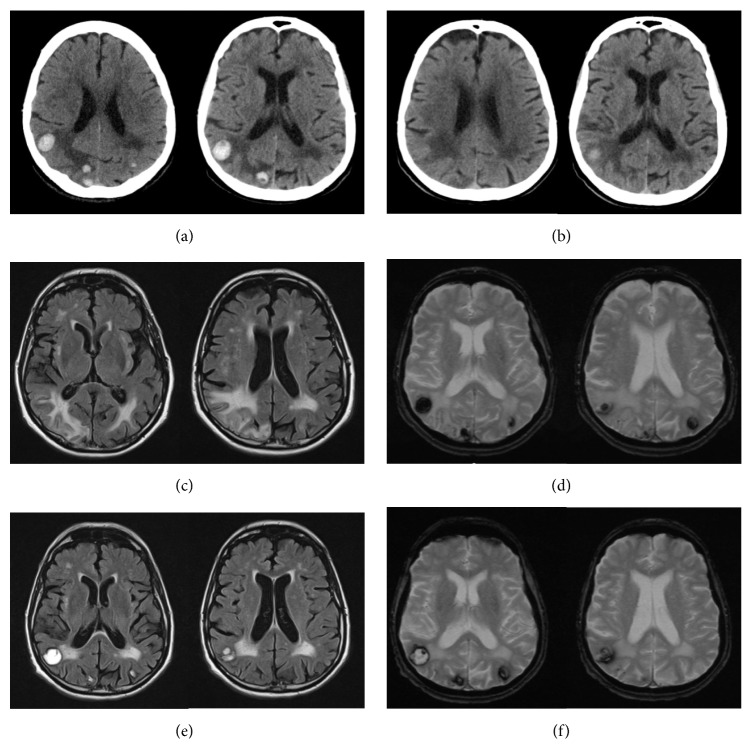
Patient 1: noncontrast brain CT-scan showing occipital bilateral hyperdense nodules, predominantly on the right side, surrounded by edema, corresponding to brain hematomas (a). Noncontrast brain CT-scan 18 days later showing regression of the size and number of hematomas with a decrease in the degree of the surrounding edema (b). MRI-scan 6 weeks later, axial Fluid Attenuated Inversion Recovery, FLAIR (c), and Gradient Echo, GRE (d), weighted sequences showing a marked susceptibility effect confirming the haemorrhagic lesions and the presence of surrounding edema. Follow-up MRI-scan 4 months later with same sequences, showing regression of the size and number of hematomas with increase in the size of the vasogenic edema in the left occipital lobe (e and f).
